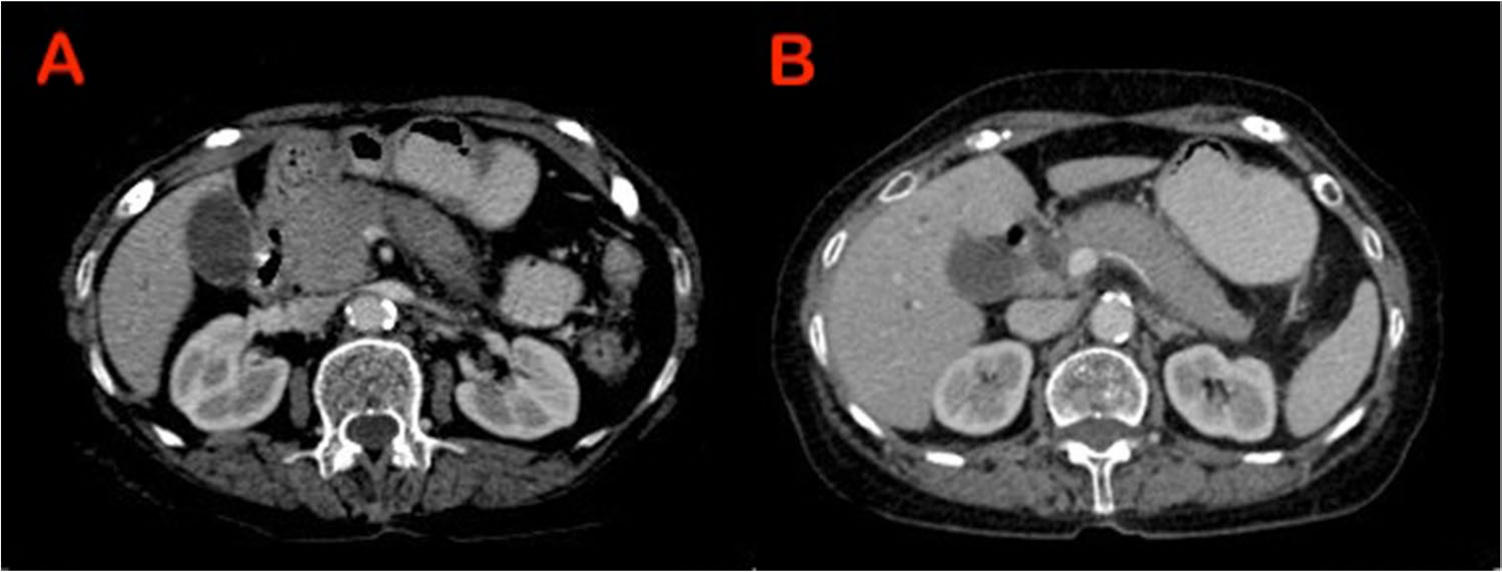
Computerised tomography (CT) showing sausage shaped enlargement of the (A) head of pancreas (B) Body and tail of pancreas - suggestive of diffuse autoimmune pancreatitis
